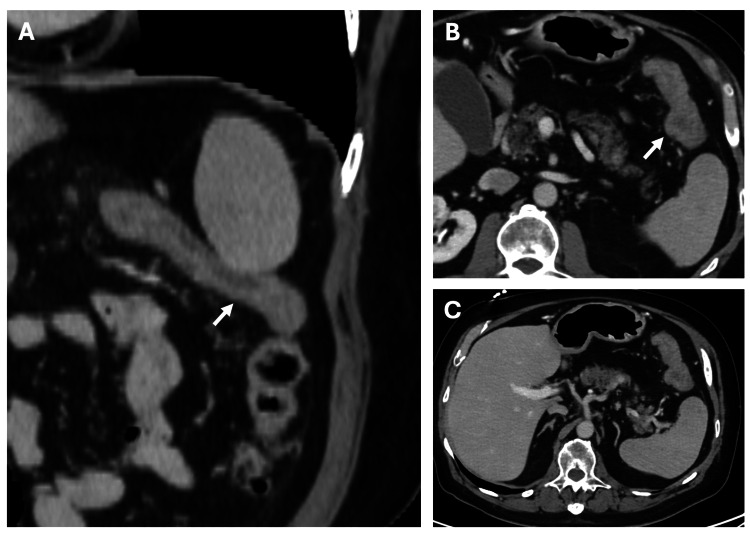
CT angiography of the abdomen and pelvis with (A) coronal and (B and C) axial views revealing diffuse wall thickening with mild pericolonic fat stranding involving the splenic colonic flexure (indicated by arrows), concerning for acute ischemic colitis.
